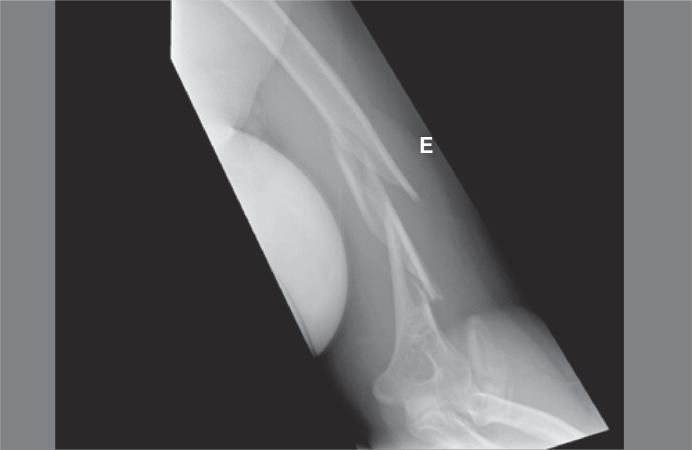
Preoperative X-Ray (AP) of patient with fracture of the distal third of the humeral shaft.
